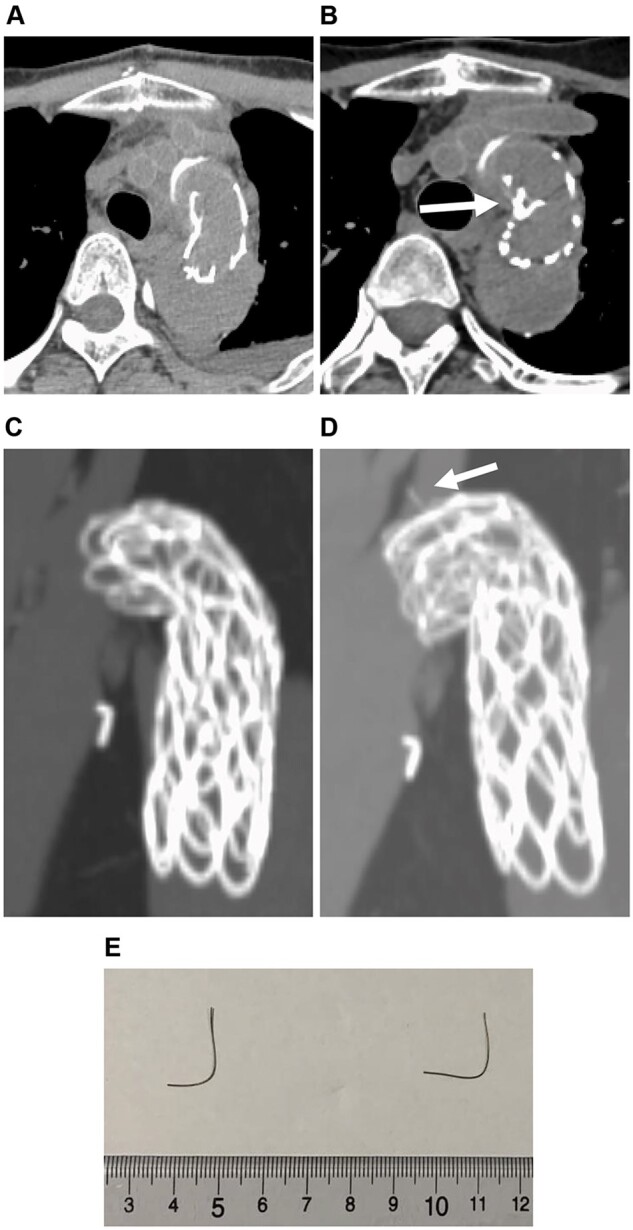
(A, C) Computed tomography scan immediately after total arch replacement. (B) Computed tomography scan showing a part of the wire that protruded into the frozen elephant trunk lumen (arrow). (D) Three-dimensional computed tomography scan showing a broken wire that protruded outward from the stent graft (arrow). (E) Each of the two picked-out metal pieces from the right common iliac artery and the left internal iliac artery.
